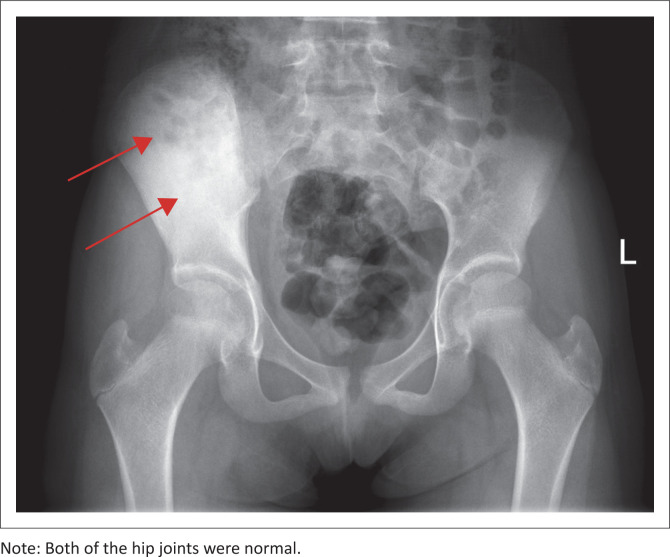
An 11-year-old-girl with chronic recurrent multifocal osteomyelitis: Initial plain radiograph of the pelvis demonstrated diffuse sclerosis of the right iliac wing (arrows).
